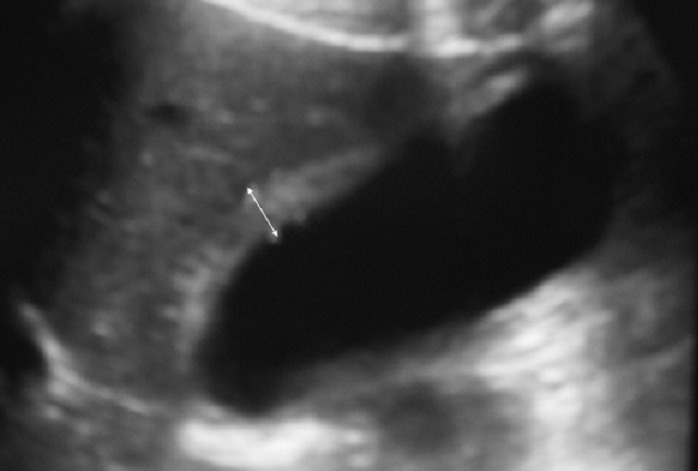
Ultrasound examination showing deformed gallbladder with a localized thickening in its wall
